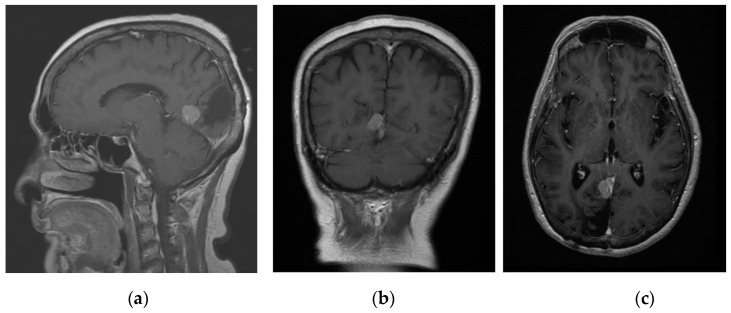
Contrasted T1 MRI: (a) sagittal, (b) coronal, and (c) axial planes showing recurrence of the lesion at the surgical bed, 1.5 cm in maximum diameter.
